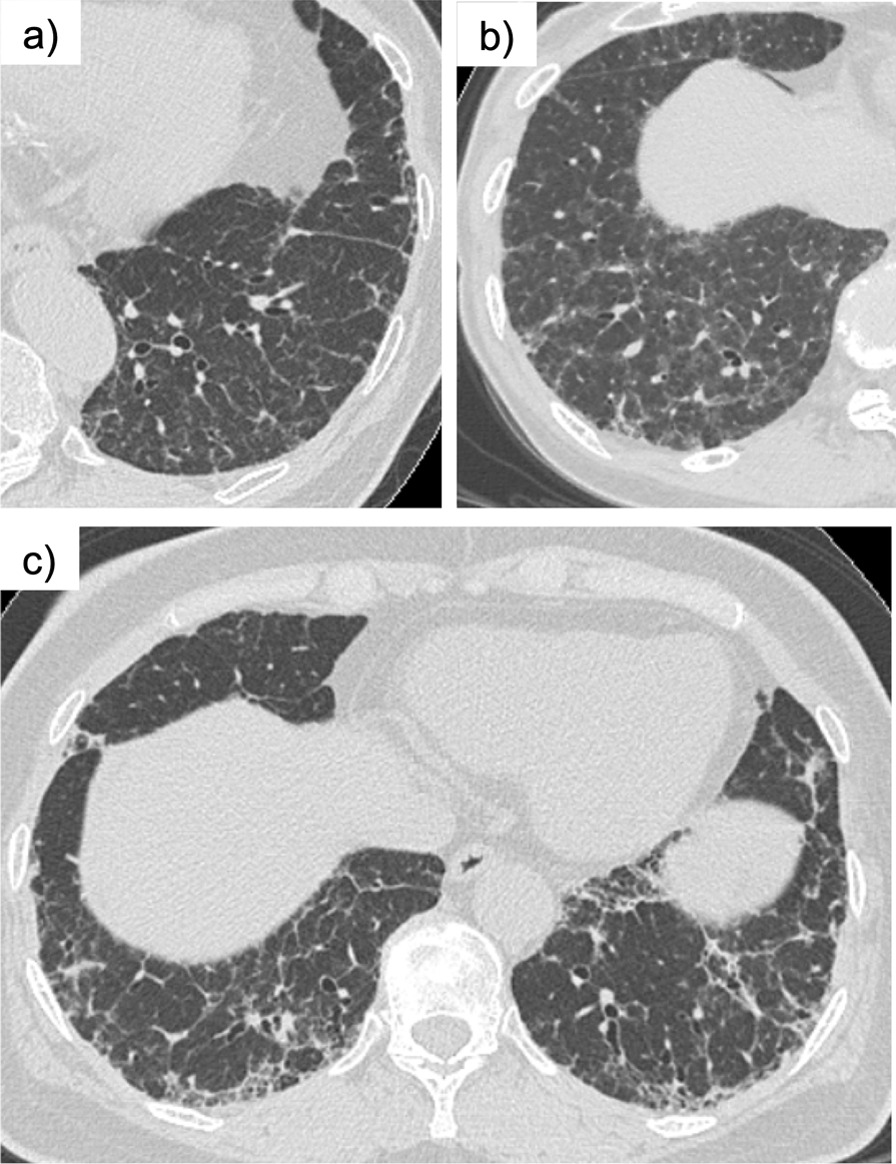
Hexagonal pattern in the HRCT. a, b Hexagonal pattern is an interlobular septal thickening expanding from the subpleural region to two or more inner layers of the secondary pulmonary lobules. c When there is subpleural collapse such that the structure of the secondary pulmonary lobules is no longer recognizable, hexagonal pattern was defined as interlobular septal thickening that expands to two or more inner layers of the secondary pulmonary lobules from the collapsed area
